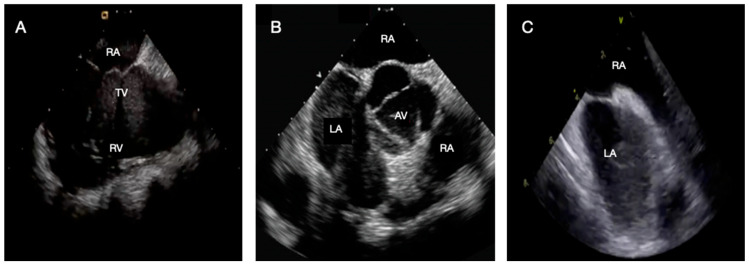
Principal ICE views from the right atrium. (A) Home view; (B) Bicaval view; (C) Aortic short-axis view. Abbreviation: ICE: intracardiac echocardiography; AV: aortic valve; LA: left atrium; RA: right atrium; RV: right ventricle; TV: tricuspid valve.
